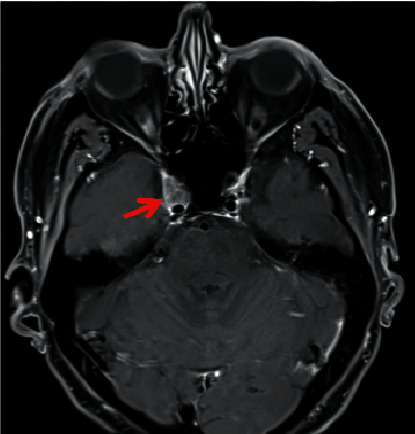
Brain MRI of Case 1. The red arrow displays metastasis within the right cavernous sinus.
